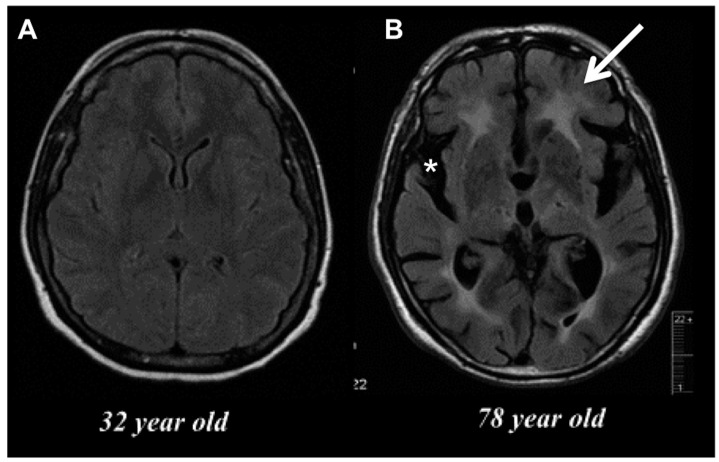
(A) Magnetic resonance imaging (MRI) of a normal 32 year old woman. There is no evidence of atrophy or white matter disease; (B) MRI of a 78 year old woman with mild cognitive impairment. There is considerable frontal temporal atrophy as seen by an enlarged Sylvian fissure (asterisks) and white matter disease (white arrow).
